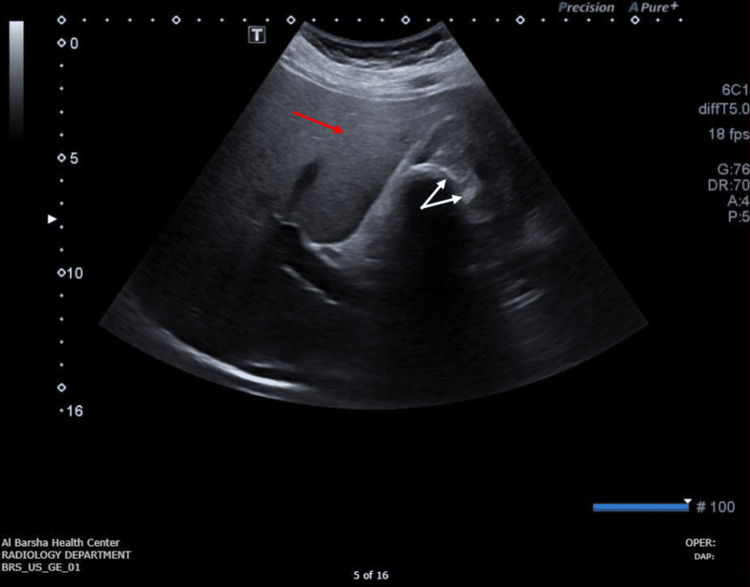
Abdominal ultrasound showing a few intraluminal gallbladder calculi, the largest measuring 2.1 X 2.5 cm with intraluminal debris and mild diffuse wall thickening represented by the white arrows. The liver is mildly enlarged (17 cm), with a bright echo pattern, smooth, regular surface, no focal lesion, and no dilated intrahepatic biliary radicals - normal hepatic veins represented by the red arrow Red arrow: liver; white arrows: gallbladder calculi Not mentioned in the figure but noted while performing the ultrasound: areas of focal fat sparing are noted within the right lobe. The portal vein is not dilated and shows normal hepatorenal flow. There is no abnormal vascularity and no pericholecystic collection. Common bile duct (CBD): This is prominent at porta (6 mm); however, the distal CBD is obscured by bowel gasses. The pancreas, spleen, and kidneys are of standard size with no abnormalities.
